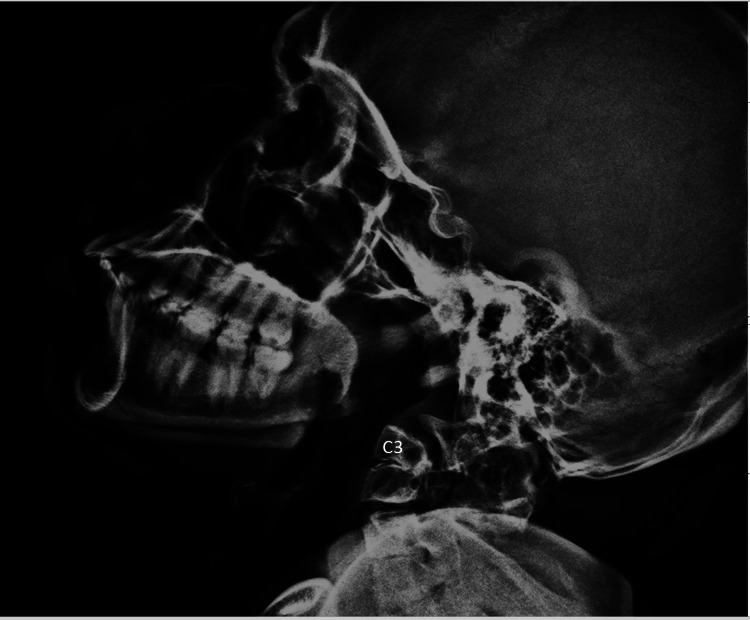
On lateral X-ray imaging, assimilation of the anterior arch with occipital bone and protrusion of odontoid process in the posterior fossa was noted. C3-C4 vertebral bodies appeared partially fused.
